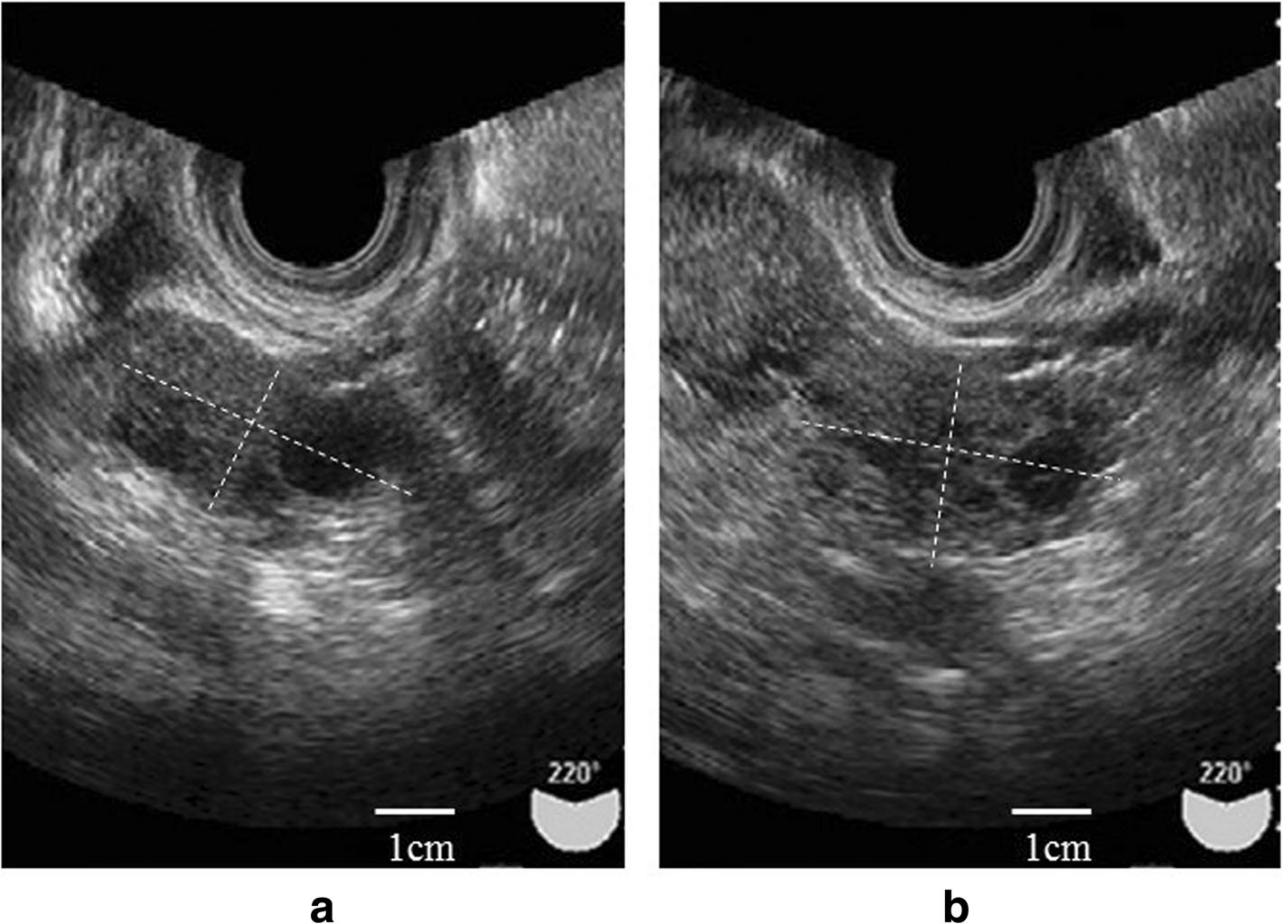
Transvaginal ultrasonograms on postoperative day 242. a Restored right ovary. b Restored left ovary
